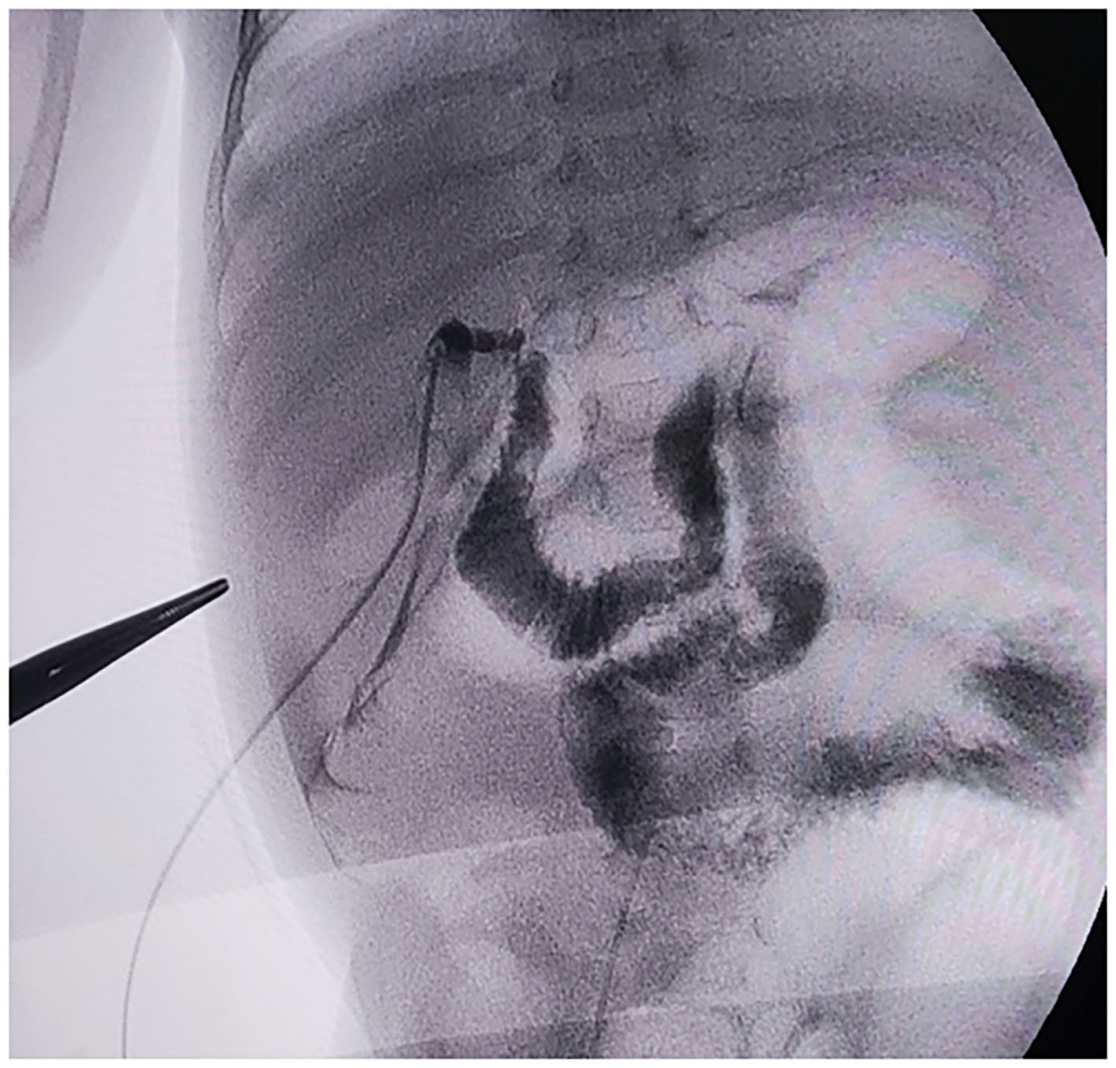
X-ray of the cholangiography during the second operation.
